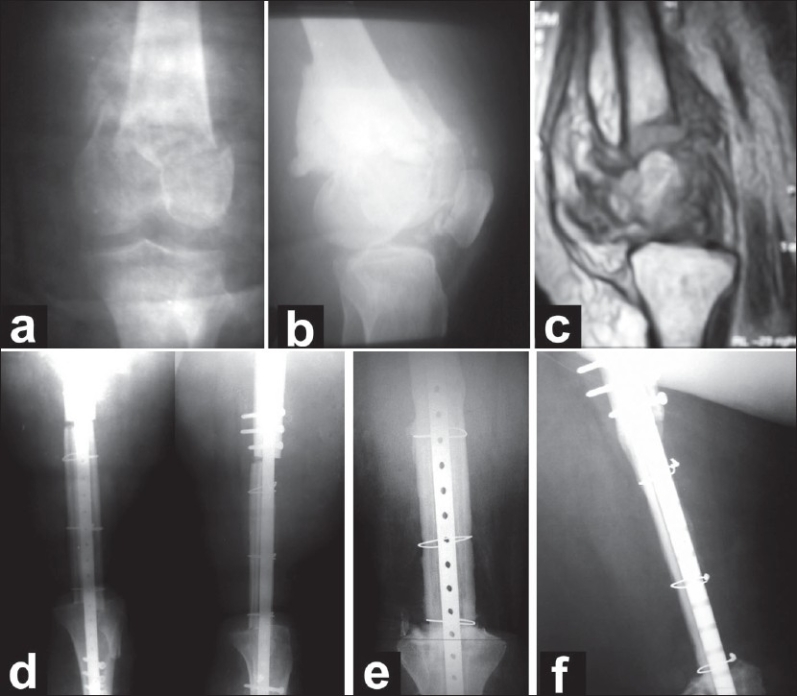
Case 1. (a and b) Preoperative X-rays showing osteolytic lesion of the distal femur with pathological fracture and bone cement. (c) MRI showing extensive soft tissue involvement and pathological fracture. (d) Immediate postoperative X-rays. (e and f) X-rays after 12 months showing full union of the graft at both the proximal and distal end.
